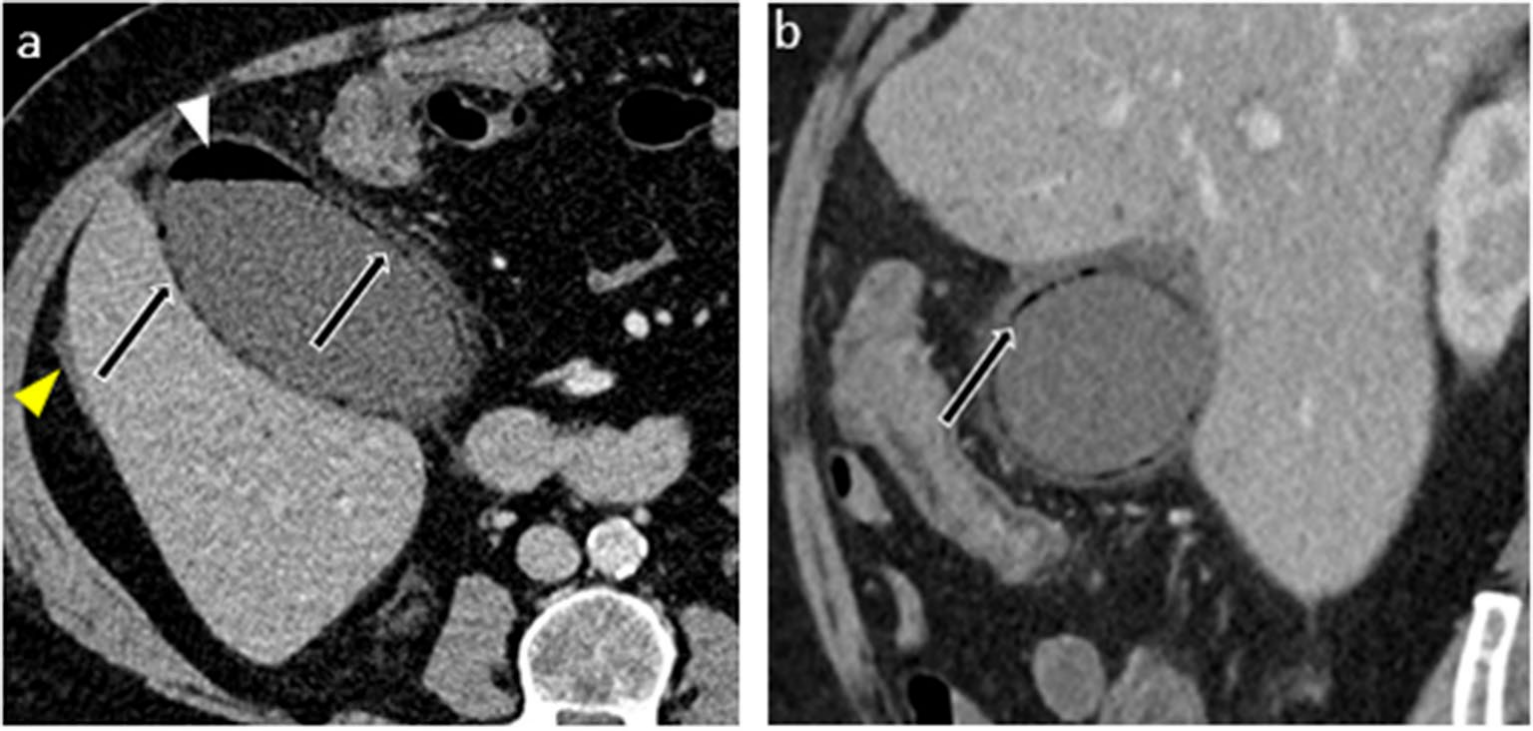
Emphysematous cholecystitis in a 67-year-old female with a history of obesity, diabetes mellitus presents to the ED via walk-in with a chief complaint of abdominal pain, worst in right upper quadrant. Axial (image a) and sagittal (image b) contrast enhanced CT demonstrates air in the wall (arrows) and in the lumen (arrowhead) of the gallbladder. There is pericholecystic stranding and trace perihepatic fluid (yellow arrowhead)
